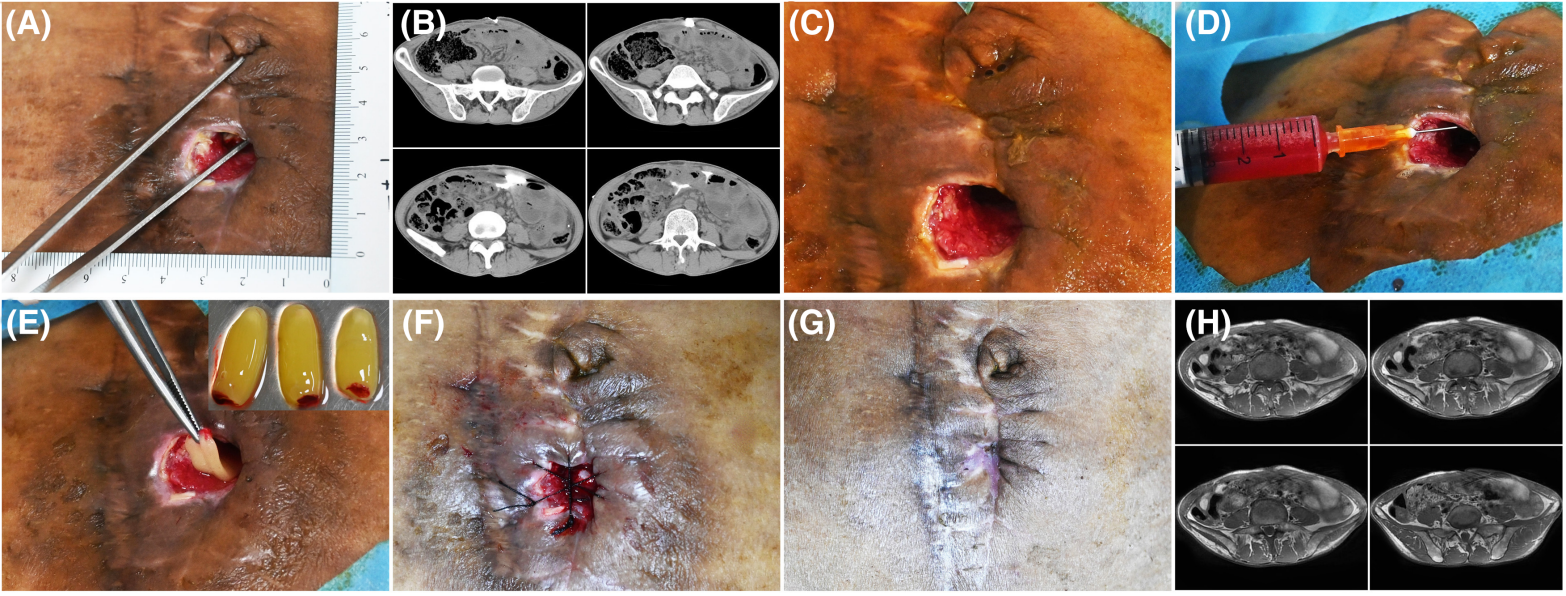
Patient 1. A 50-year-old man developed an abdominal fistula after intestinal adhesion lysis for 2 months. (A) Baseline. The depth of the sinus tract was checked with sterile surgical forceps. (B) The enhanced computed tomography showing the abdominal fistula. (C) Pre-PRP treatment. The wound was clean after debridement. (D) Treatment day. The wound bed was injected with PRP. (E) Treatment day. The fistula was filled with PRF. (F) Treatment day. The wound was sutured with silk thread. (G) 14 days after the treatment. (H) 16 days after the treatment, MRI showing the healing of abdominal fistula.
